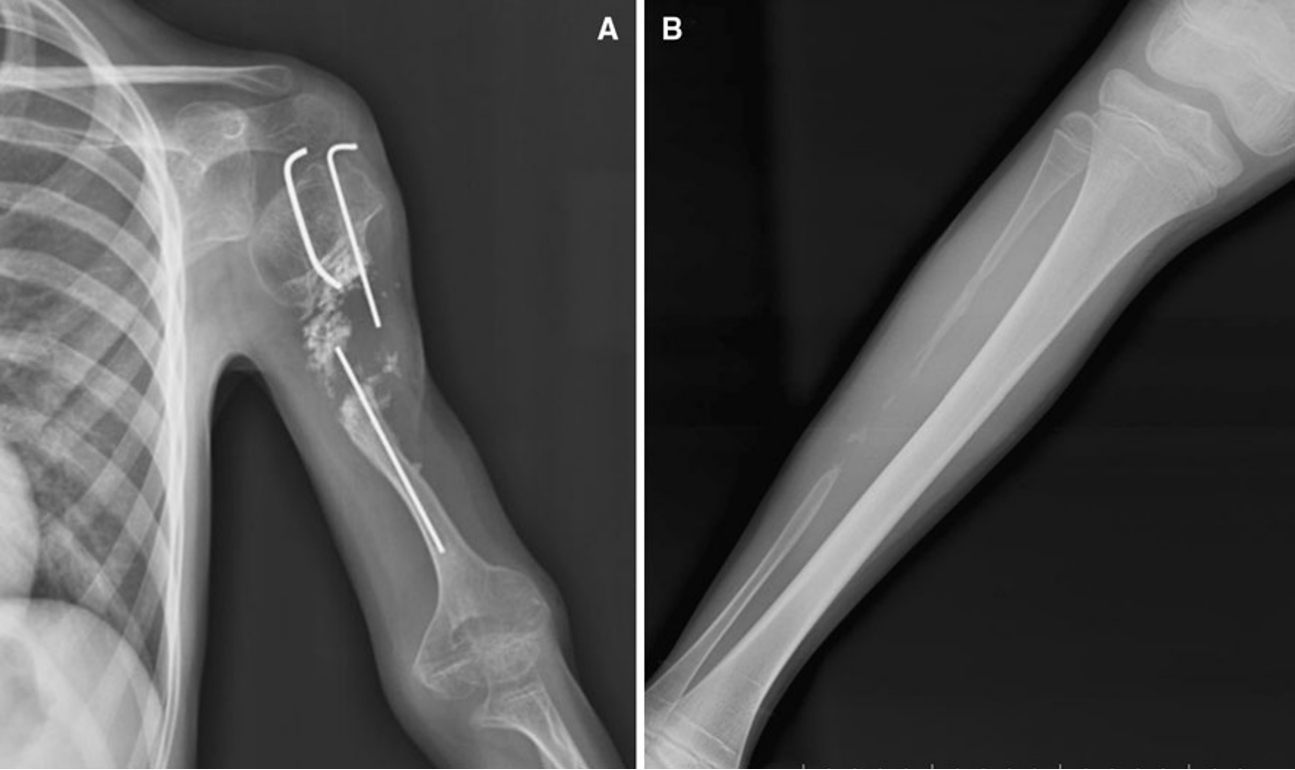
At the end of 12th month follow-up, anteroposterior radiograph (a) shows failure of the fibular graft fixation and nonunion of the humerus. Plain radiograph of the *right leg* (b) shows new bone formation in the donor side without any angular deformity
